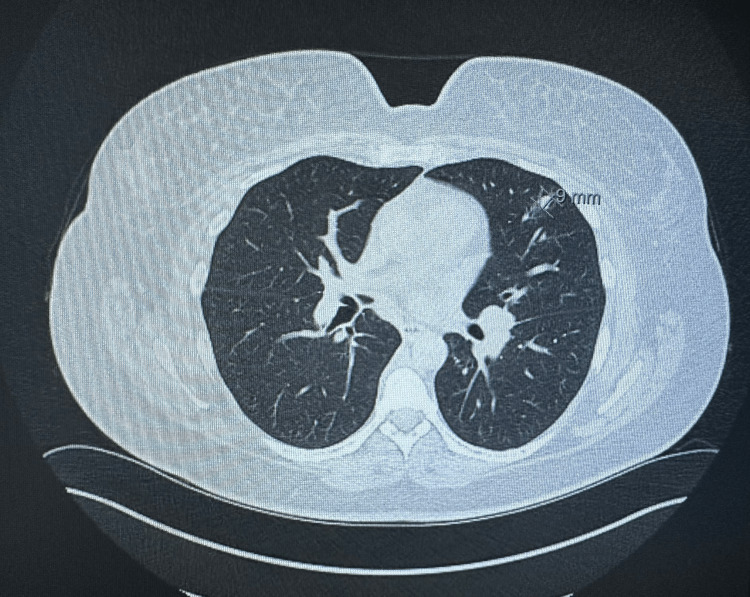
Computed tomography of the chest showing a pulmonary nodule of 9 mm in size.
